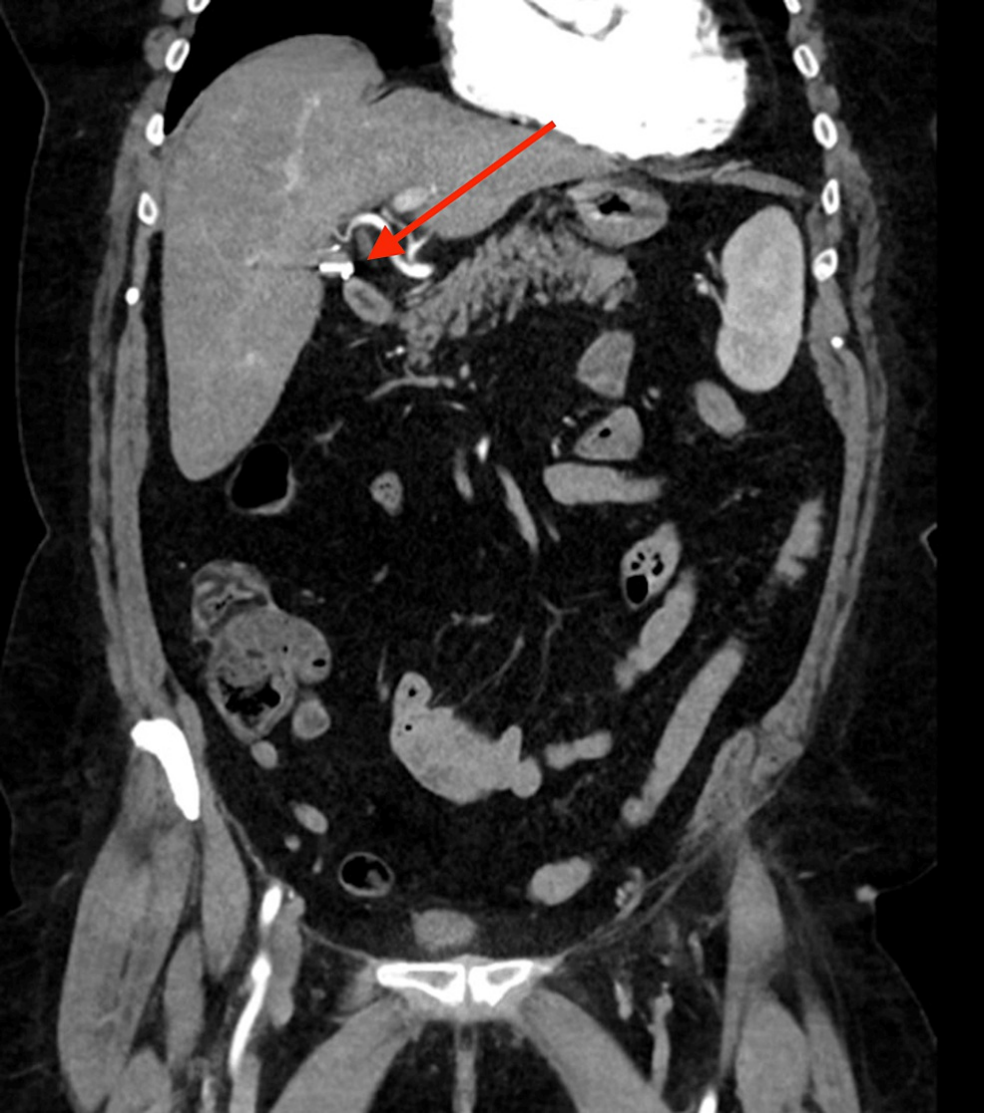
Computed tomography imaging of the patient’s first presentation demonstrating clips present at the cystic duct stump, as identified by the red arrow
